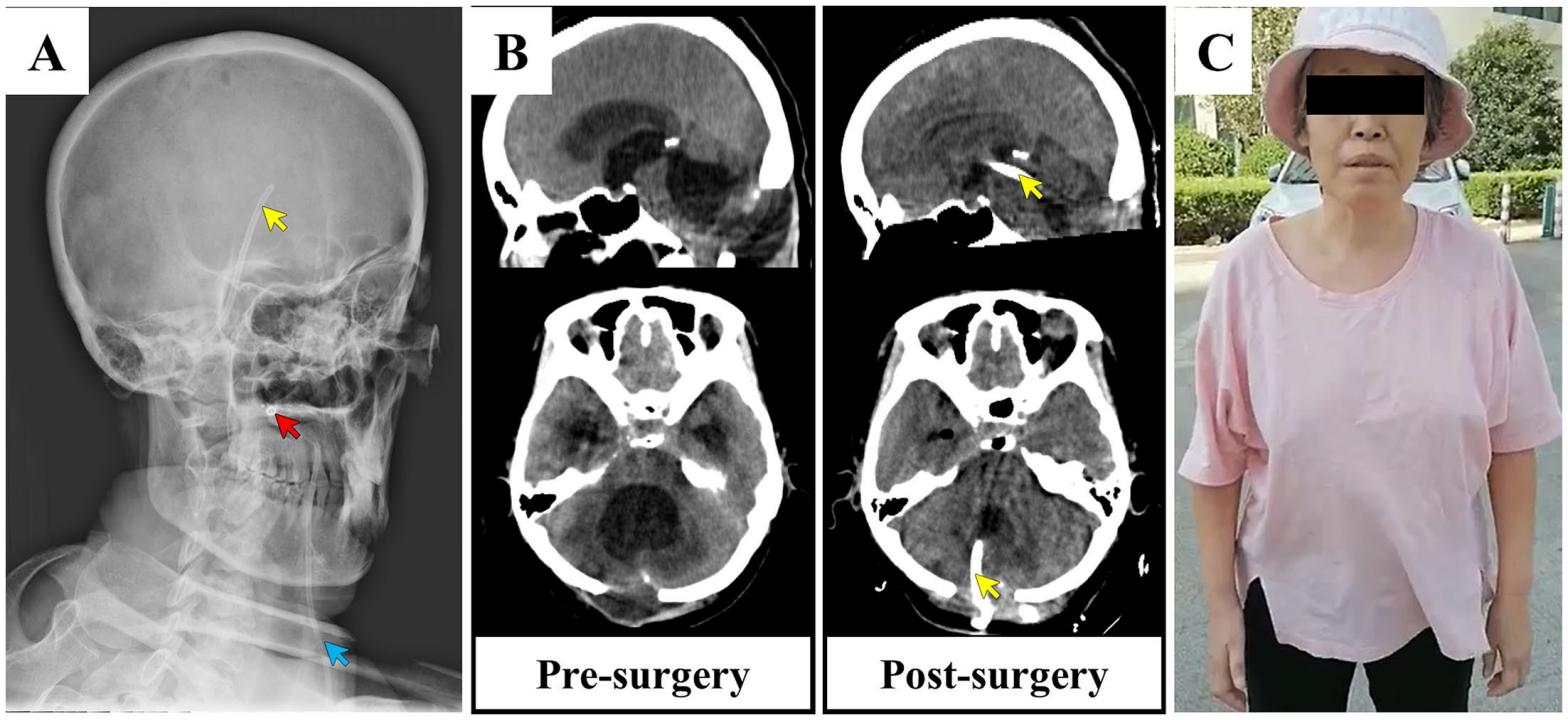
Post-operative images of the patient and CT comparison before and after surgery. (A) Postoperative X-ray image revealing the shunt tube located within the ventricle (indicated by the yellow arrow) and the valve positioned beneath the skin (marked by the red arrow). (B) Comparative CT images taken before and after surgery, showing alterations in the ventricular system. The postoperative CT scan indicates a reduction in the size of the temporal horn of the third ventricle, the fourth ventricle, and the lateral ventricle. The yellow arrow points to the location of the intraventricular shunt tube. (C) Follow-up photographs of the patient taken one year after discharge, providing visual evidence of the patient’s condition and recovery. CT, computed tomography.
